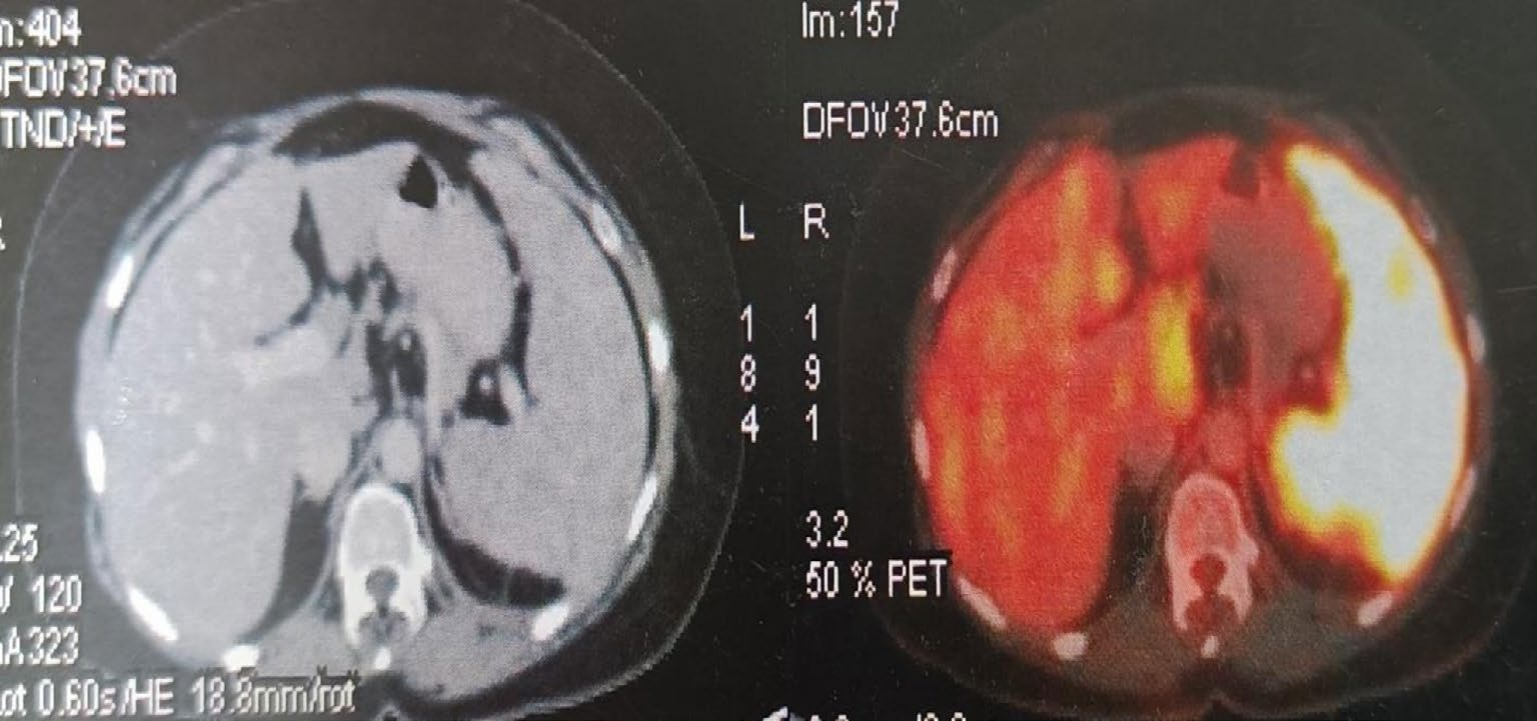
Transverse section on PET‐CT showing increased FDG uptake in liver, spleen, and multiple abdominopelvic lymph nodes.
